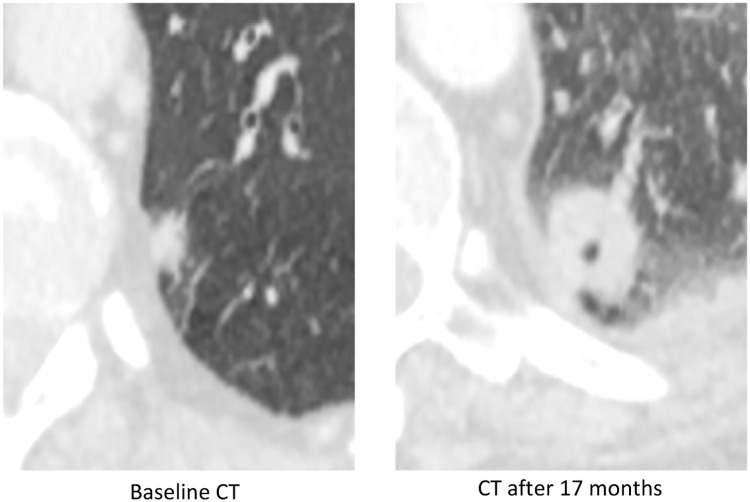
Example of a malignant nodule of 10 mm of the LLL in axial slices on the baseline scanner. A recommendation of follow-up after 3 months was made but was not implemented. A CT scan performed 17 months later out of context for suspected pulmonary embolism revealed a significant increase in the nodule, ultimately corresponding to an adenocarcinoma confirmed by lung biopsy. *The use of AI software could potentially have reduced this diagnostic delay by increasing the need for follow-up, given the high AI score of the nodule on the CT Baseline (IA Score = 94.52)*.
